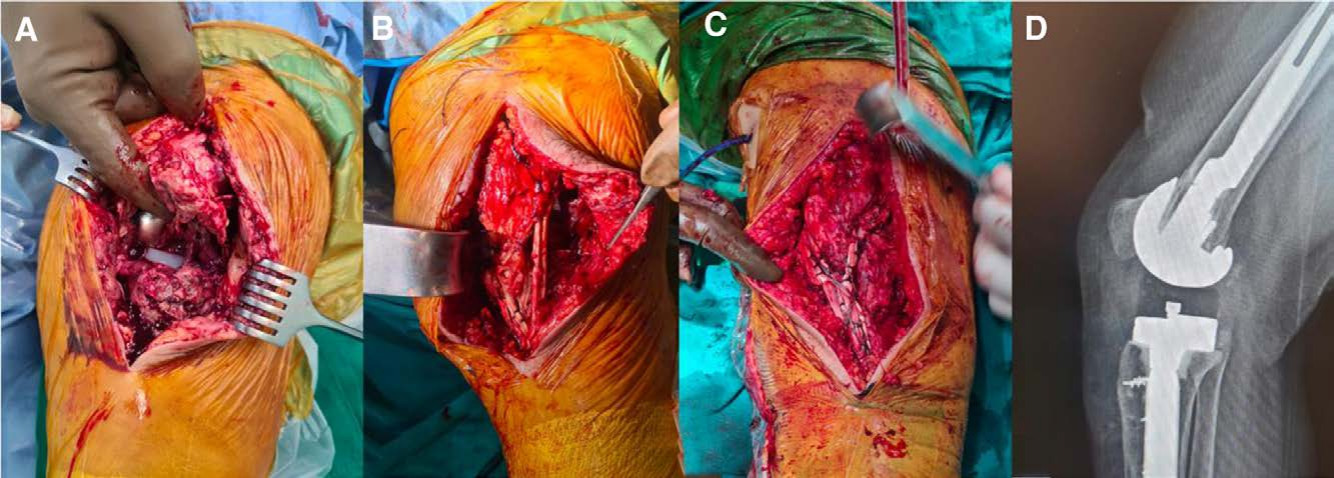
Intraoperative images of the patient. (A) Intraoperative patellar tendon tear image. (B) Image after the graft tendons are threaded and augmented. (C) Image after PEEK anchor placement and total reconstruction is completed. (D) Lateral view of postoperative plain radiograph.
